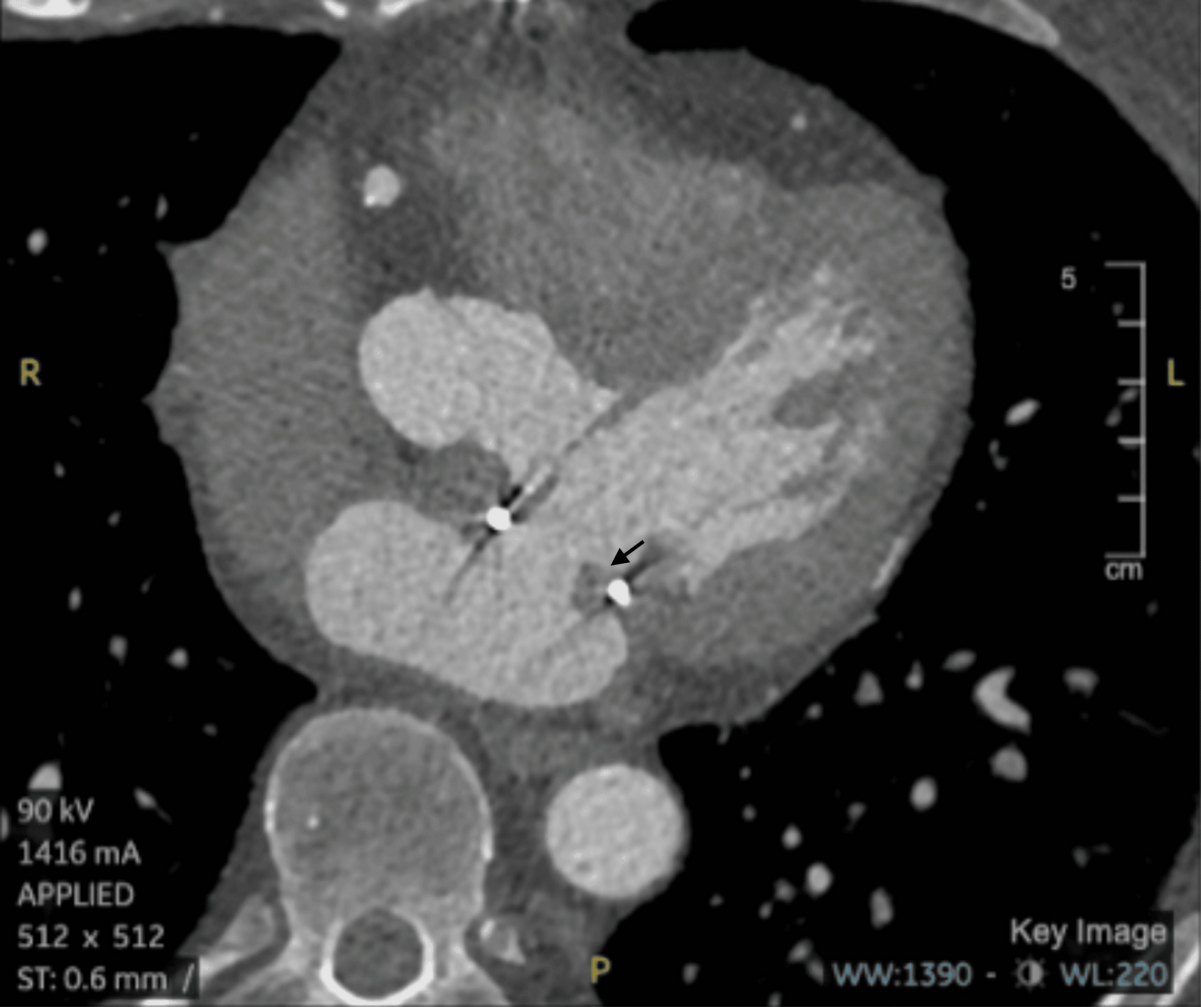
Cardiac Computed Tomography Imaging of the Prosthetic Mitral Valve Axial computed tomography image demonstrating abnormality around the prosthetic mitral valve repair, including early pannus formation and possible thrombus or vegetation. Image was obtained from the patient with their permission.
